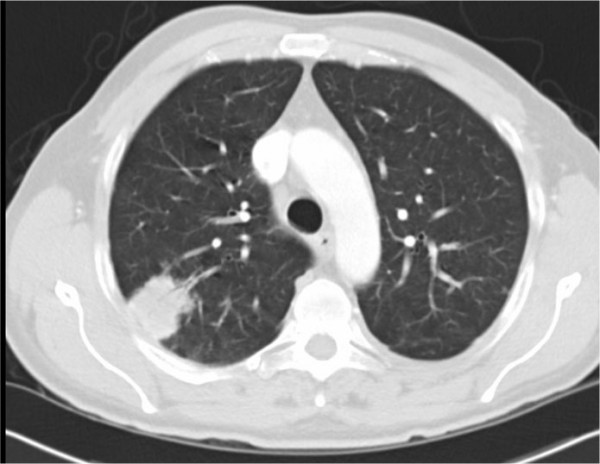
Computed tomography (CT) imaging of the chest.
